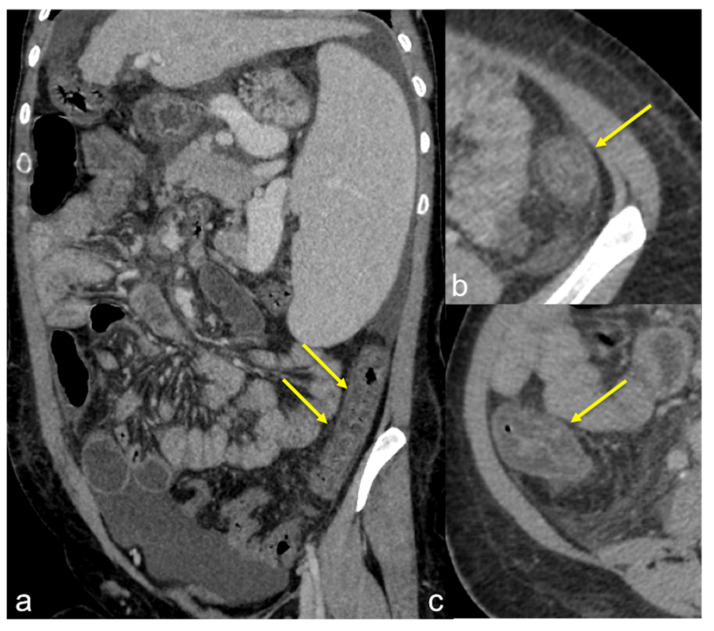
Ischaemic colitis in a cirrhotic patient. Enhanced CTA scan in portal venous phase in coronal-oblique (a) and axial (b,c) views. Note the typical colonic involvement (a–c_a;b;c;b;c;d;d;e;f;d;e;f;a;b;c;a;b;c;d_) arrows) with stratified wall thickening with submucosal oedema, mucosal hyperaemia and adjacent mesenteric stranding.
